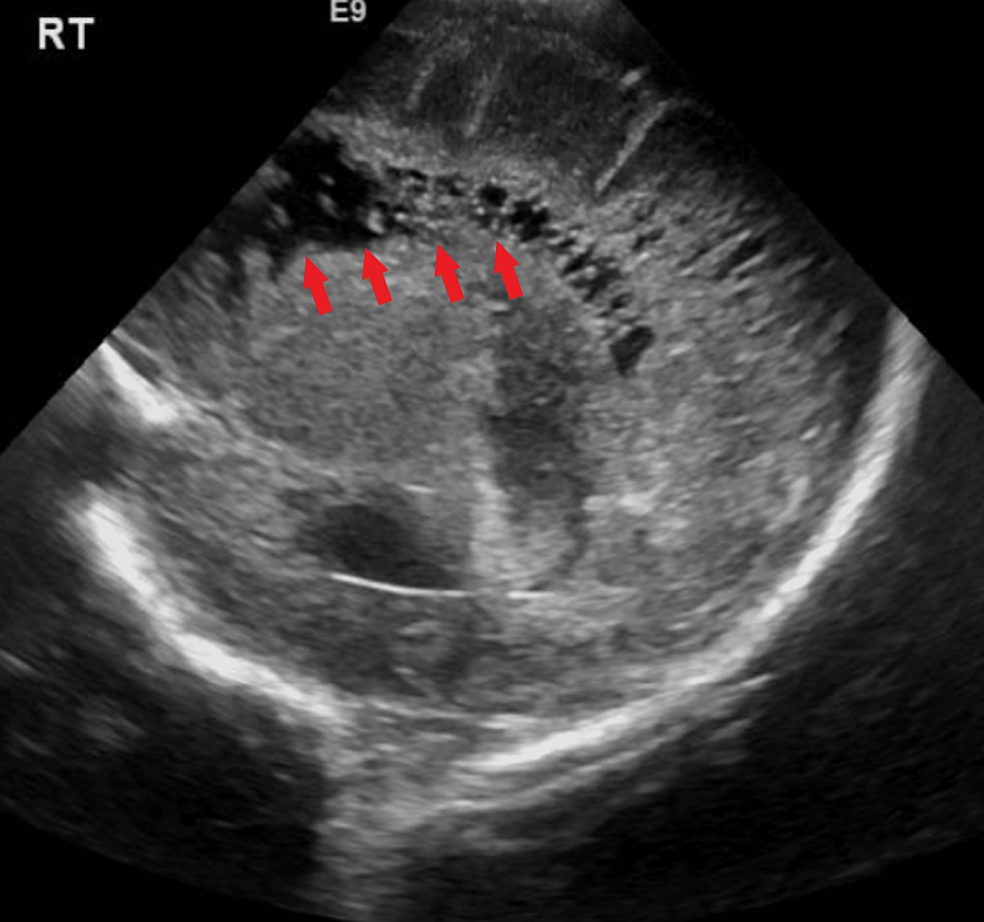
Transcranial ultrasound in the sagittal plane demonstrating cystic lesions in the distribution of the right MCA on postoperative day 21. MCA: middle cerebral artery Multiple bilateral cystic foci involving the periventricular white matter were noted.
